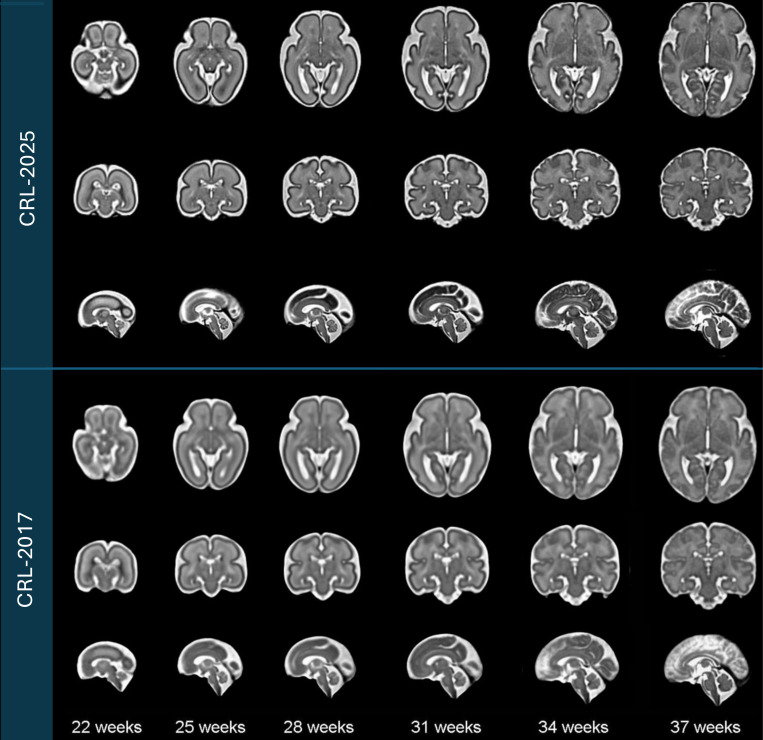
Comparison of spatiotemporal fetal brain MRI atlases ( CRL-2025 vs. CRL-2017) at six representative gestational ages: 22, 25, 28, 31, 34, and 37 weeks. Axial, coronal, and sagittal views are presented for each atlas at each age.
